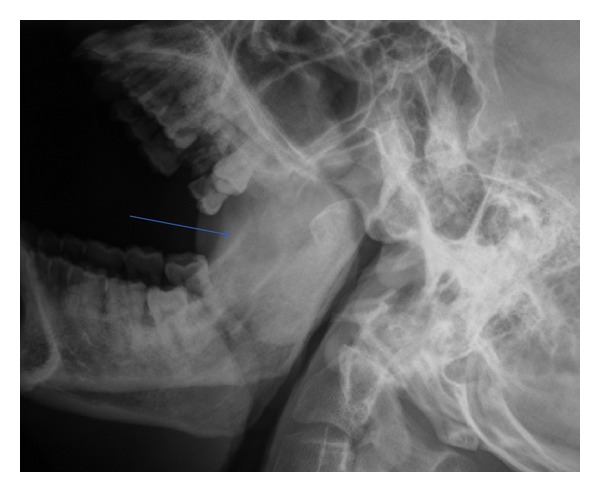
Plain radiograph of soft tissue of nasopharynx lateral view showing well defined round mass (marked by thin arrow) in the oropharyngeal region with no calcification or cavitation and causing significant narrowing of oropharynx.
